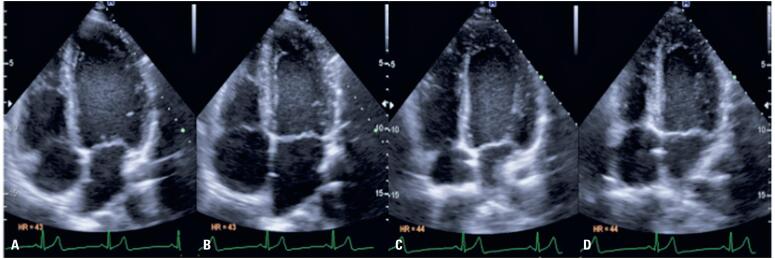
Comparison of apical four-chamber images between the conventional and prone positions. (A, B) Images obtained with the patient in the conventional position. (C, D) Images obtained with the patient in the prone position. Images A and C were obtained during diastole, and images B and D during ventricular systole
